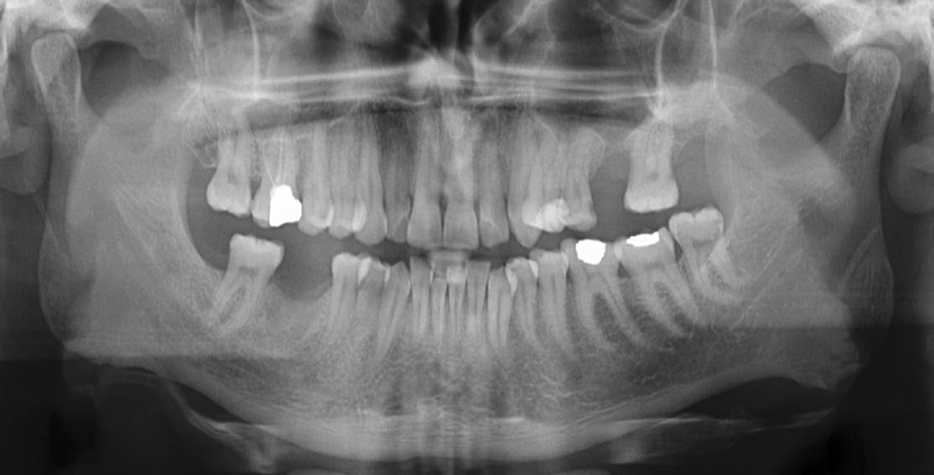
Six-month post-operative panoramic radiograph showing the defect filled new bone.
